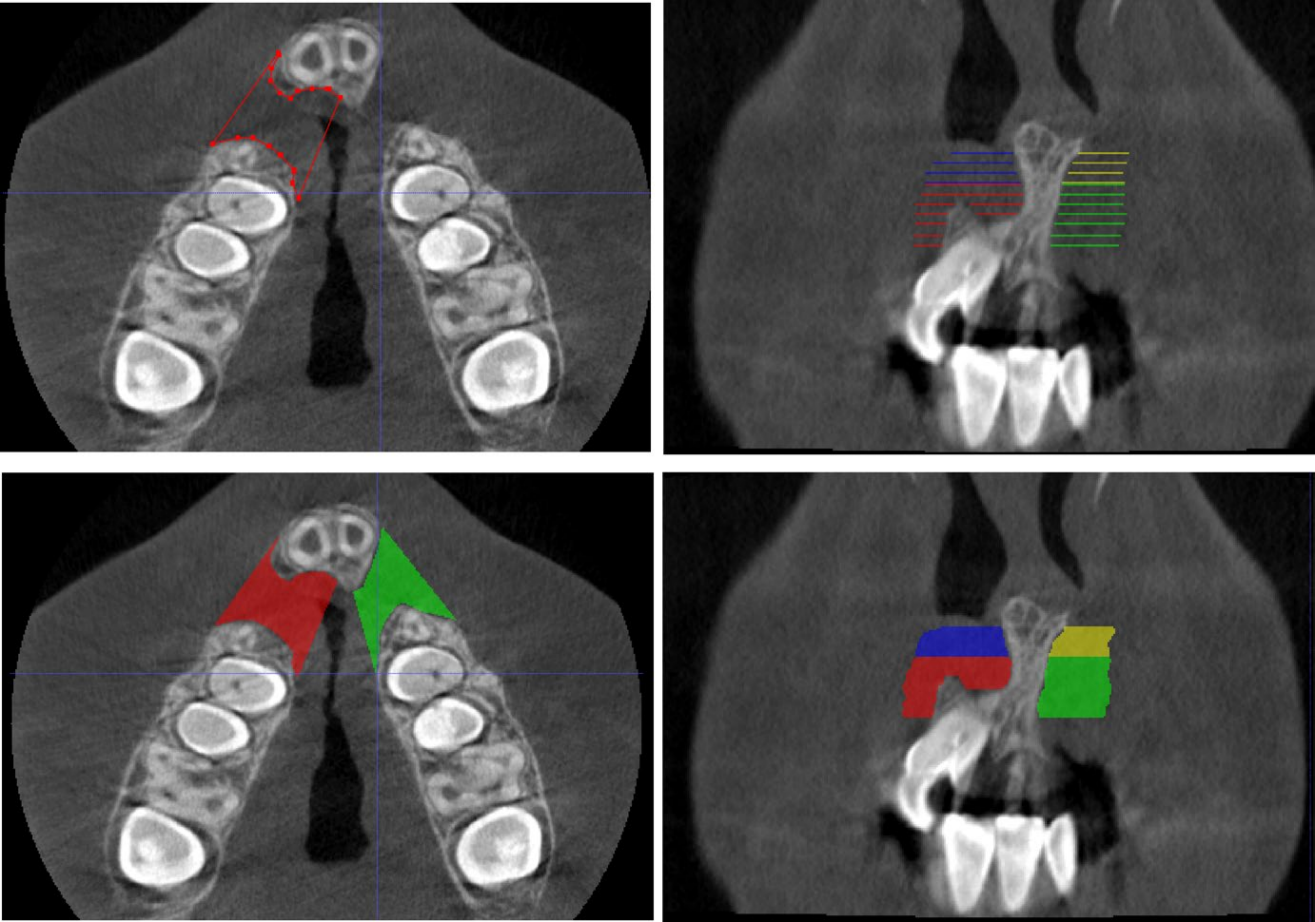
Manual segmentation of the cleft area at different levels performed every mm in Cone‐beam Computed Tomography scan using the software ITK‐SNAP (upper images). Control of the accuracy of the segmented cleft volumes after interpolation of the superior and inferior volumes in Cone‐beam computed tomography scan using the software ITK‐SNAP (lower images). The blue and yellow areas are the superior part of the cleft, while the red and green are the inferior part.
